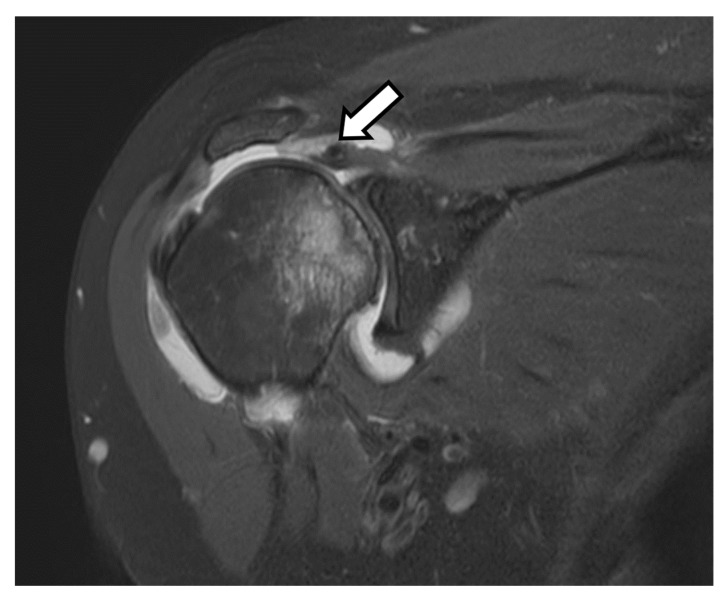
Coronal T2-weighted fat-saturated magnetic resonance (MR) image shows full thickness superior cuff tear with tendon retraction close to the level of the glenoid (arrow). Bone marrow edema and developing cartilage loss along the humeral head is consistent with developing cuff tear arthritis.
